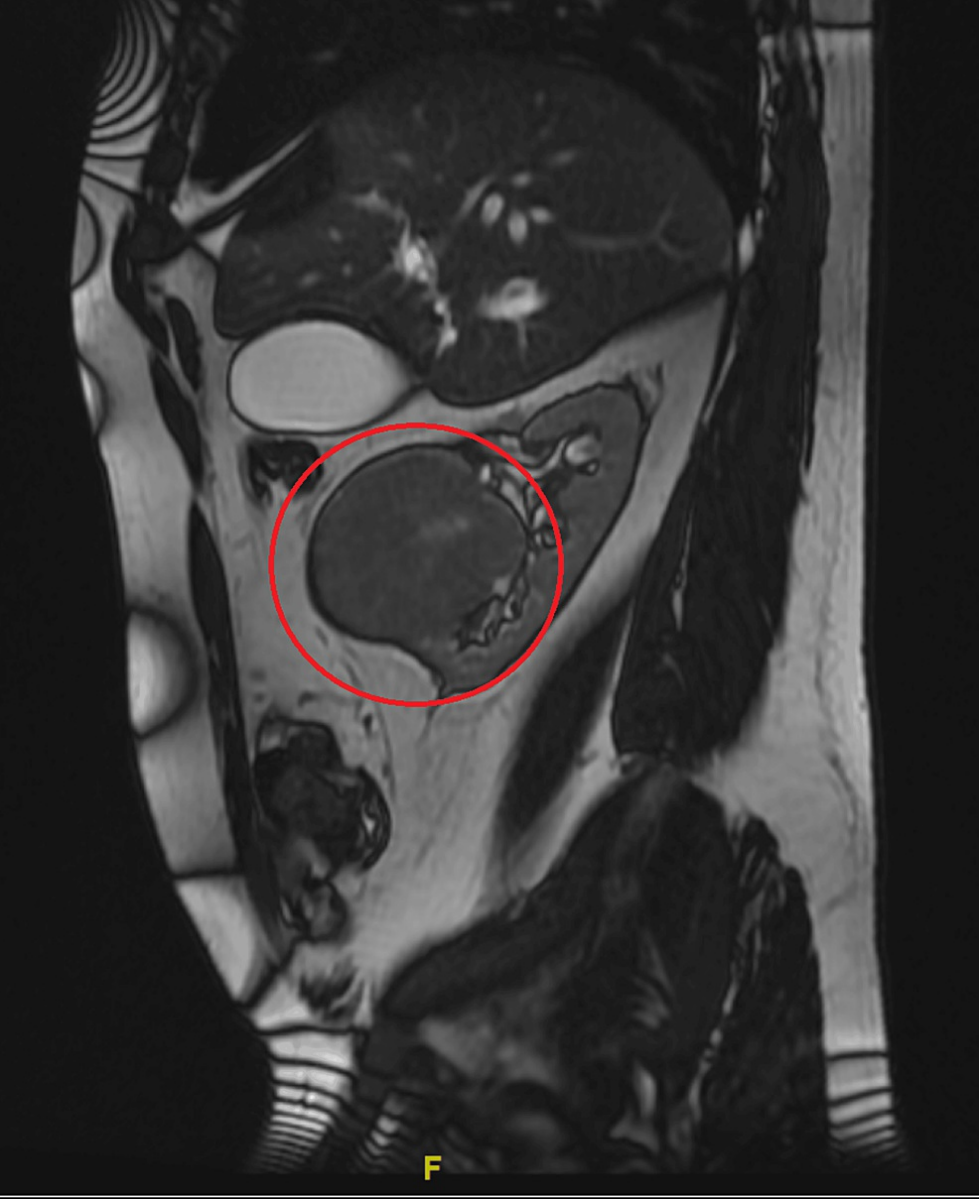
Renal cell carcinoma of the right kidney captured on magnetic resonance imaging sagittal view prior to nephrectomy procedure (red circle).
